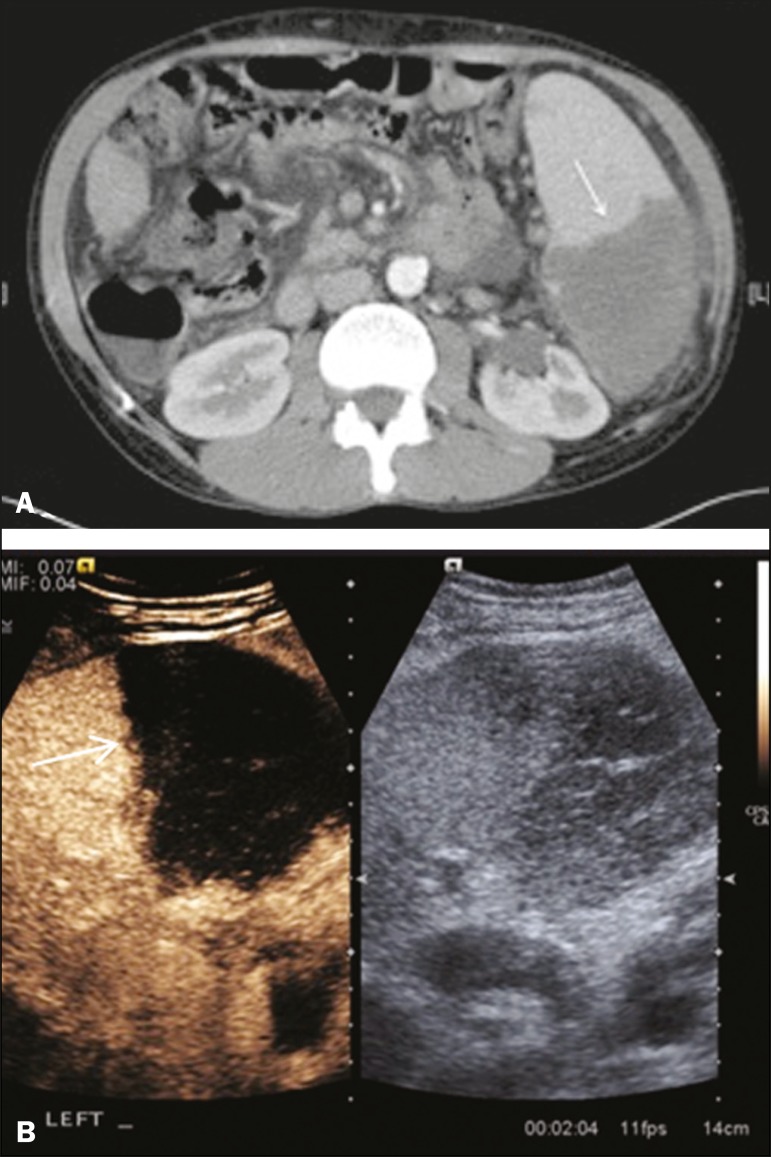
Splenic infarction. A: Contrast-enhanced CT scan, in a
